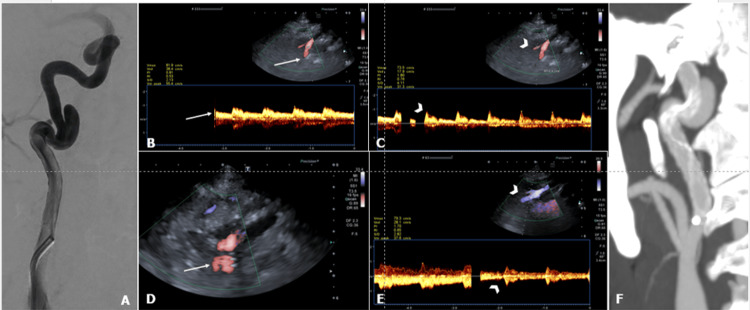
A 53-year-old man with a right high cervical ICA pseudoaneurysm. (A) Diagnostic angiography demonstrated an 8 mm wide neck pseudoaneurysm from the anteromedial high cervical internal carotid artery (ICA) wall. (B-C) Color Doppler ultrasound (US) with a transcranial probe confirmed a pseudoaneurysm with irregular arterial flow (white arrowhead in C) compared with flow from the adjacent high cervical ICA (white arrow in B). (D-E) Three-month follow-up with a transcranial probe US, demonstrating the presence of stent meshes and color signal outside the stent (arrow) along the ICA curvature; pseudoaneurysm appeared vascularized (arrowheads in E). (F) CT angiography confirmed the presence of a pseudoaneurysm with partial stent apposition on the carotid walls.
